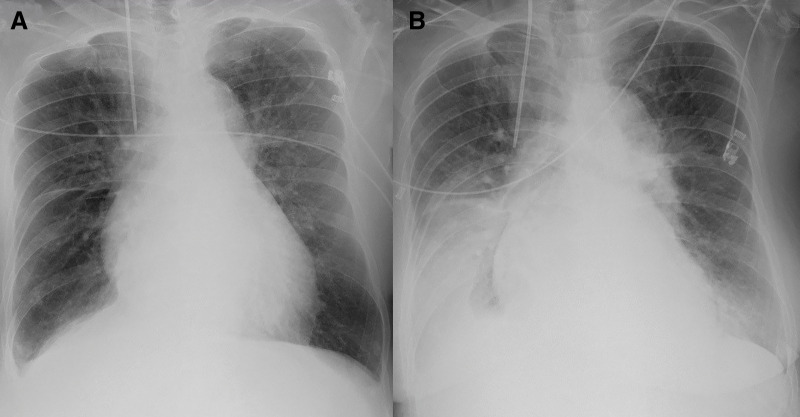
Chest radiograph findings. Chest radiograph obtained on day 5 after transfer (B) shows worsening pulmonary congestion compared with that taken at the time of transfer (A).
